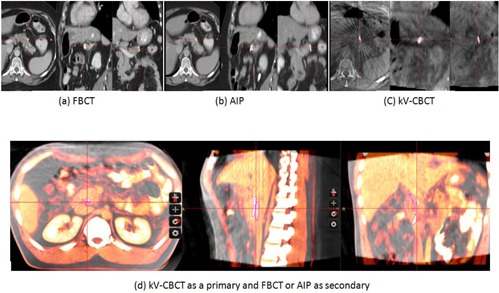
Fiducial markers shown on (a) FBCT, (b) AIP, and (c) CBCT, and (d) bony alignment without rotation correction between CBCT as the primary CT and FBCT or AIP as the secondary CT.
